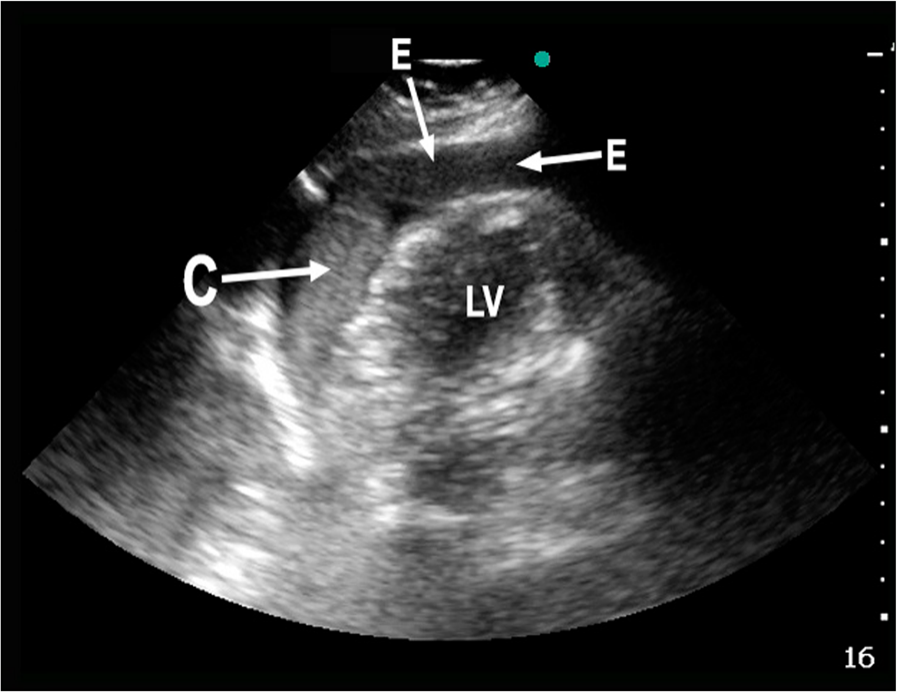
Reversed apical 4-chamber view – pericardial effusion. Pathological reversed apical 4-chamber view (the transducer is rotated 180 degrees compared to the classical apical 4-chamber view) from patient with aortic dissection and pericardial effusion. E = Effusion (blood), C = Clot, LV = Left Ventricle.
